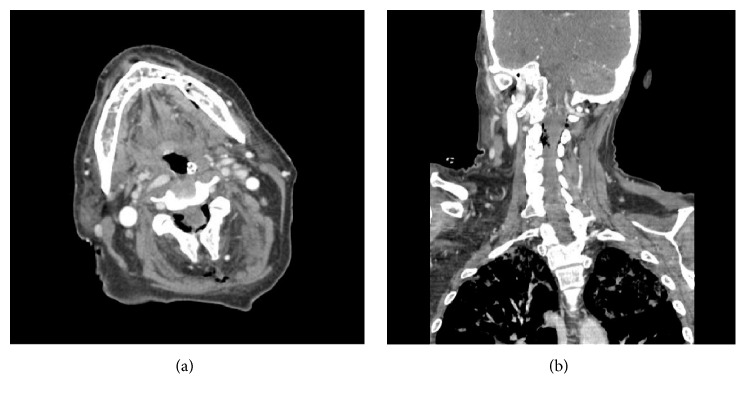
(a) CT scan shows no fluid collection or abscess. However, air is evident in the marrow on left side of the mandible. (b) CT scan showed air in the epidural space in cervical region.
